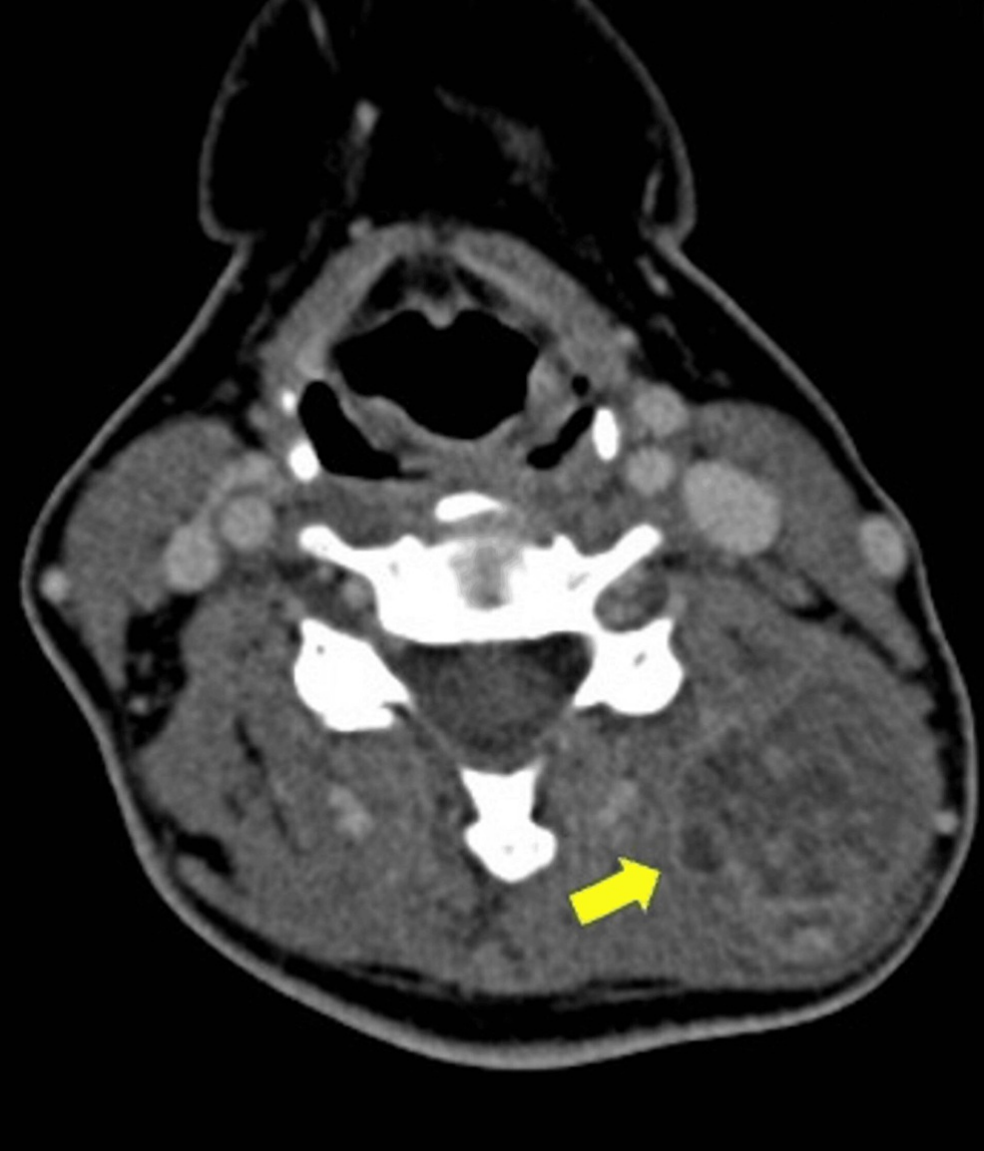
Contrasted CT scan of the neck showing a well-defined, heterogeneous, enhancing lobulated mass in the left posterior triangle of the neck measuring 3.6 x 3.2 x 4.6 cm (yellow arrowhead)
